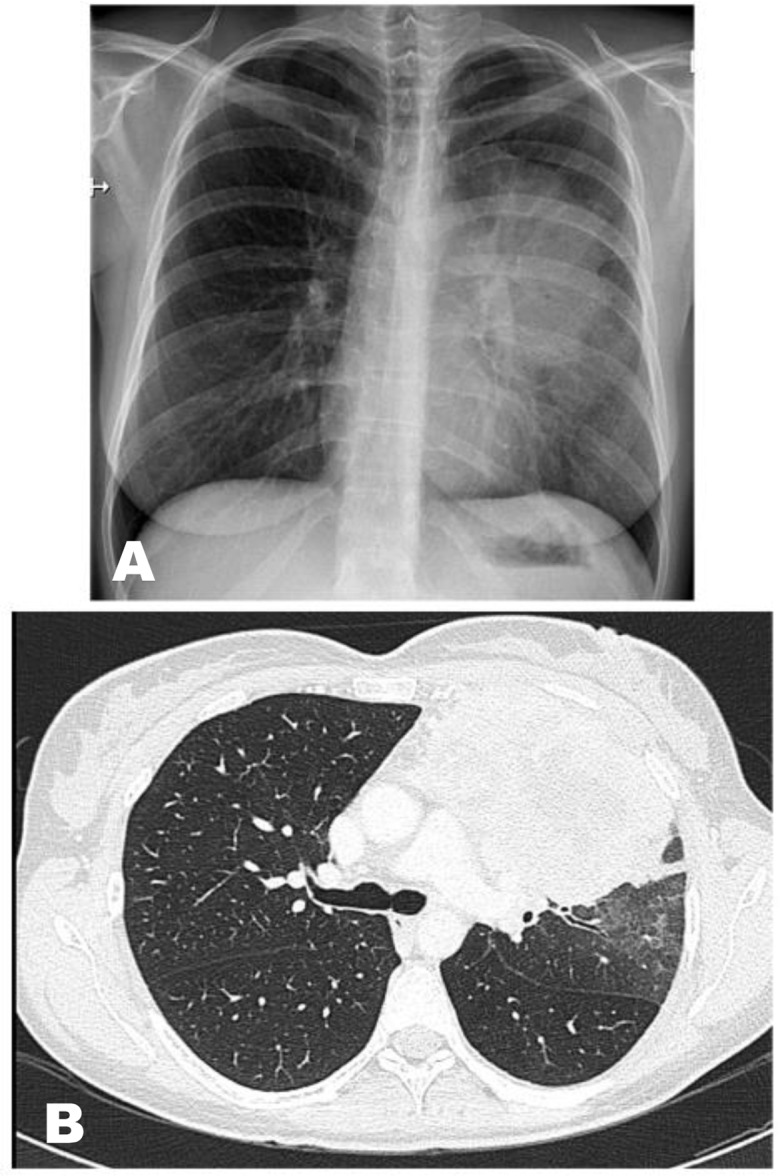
A) Chest X ray: left sided perihilar opacity, B) Computed tomography of thorax: left upper lobe solid mass
